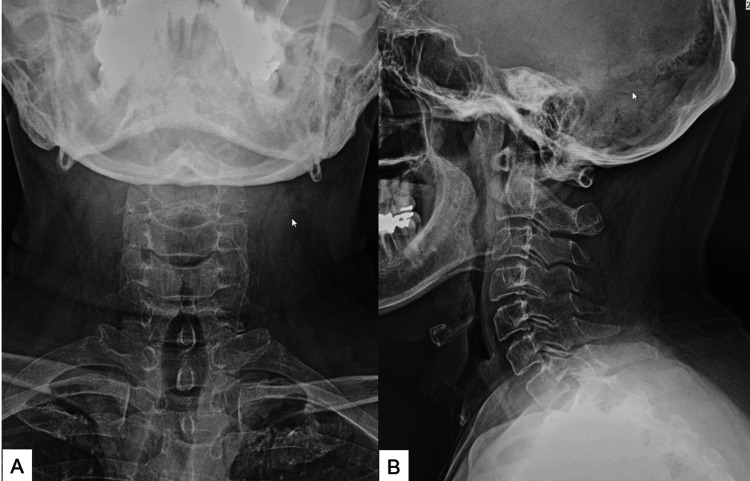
Preoperative roentgenogram: (A) anteroposterior roentgenogram and (B) lateral flexion roentgenogram. The sagittal canal width was increased at the C5 level (20.3 mm).
